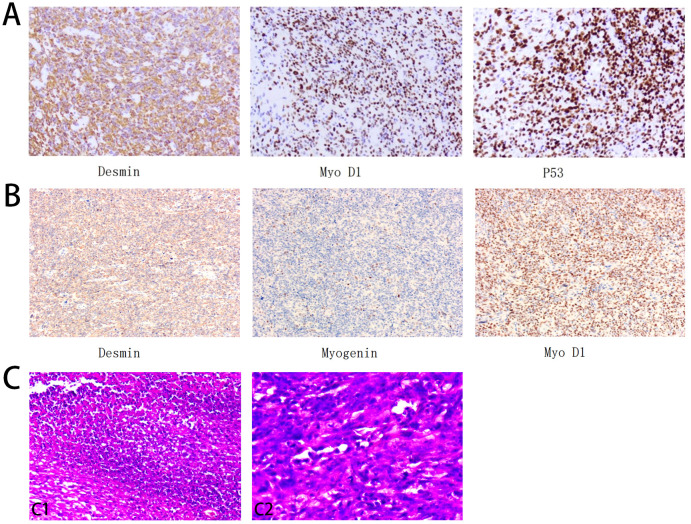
One month after the local excision of the tumor on the left lower limb, the enhanced MRI results of the left lower limb are as follows: (A) TW2-TSE signal; (B) SETTR-TSE-SENSE signal; (C) ET1W-SPIR-SENSE signal; (D) TW2-TSE signal.
